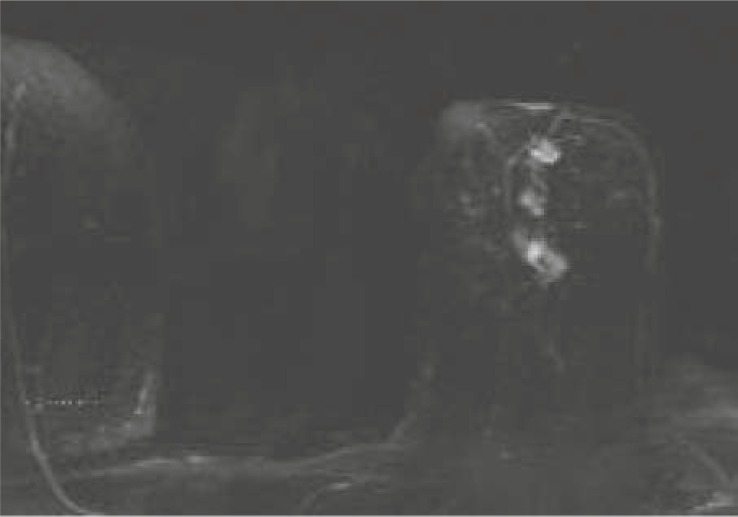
71-year old woman, MR mammography performed in the other institution. Axial T1-weighted subtracted image after Gadolinium injection (2nd minute) shows 3 round masses in her left breast, with spiculated margins, rim like enhancement, intermediate signal intensity on T2-weighted images. Kinetic curve shows fast initial enhancement with post-initial wash out. Lesions were categorized as BI-RADS 5. Pathologic diagnosis through MRI-guided vacuum-assisted biopsy was invasive lobular carcinoma.
